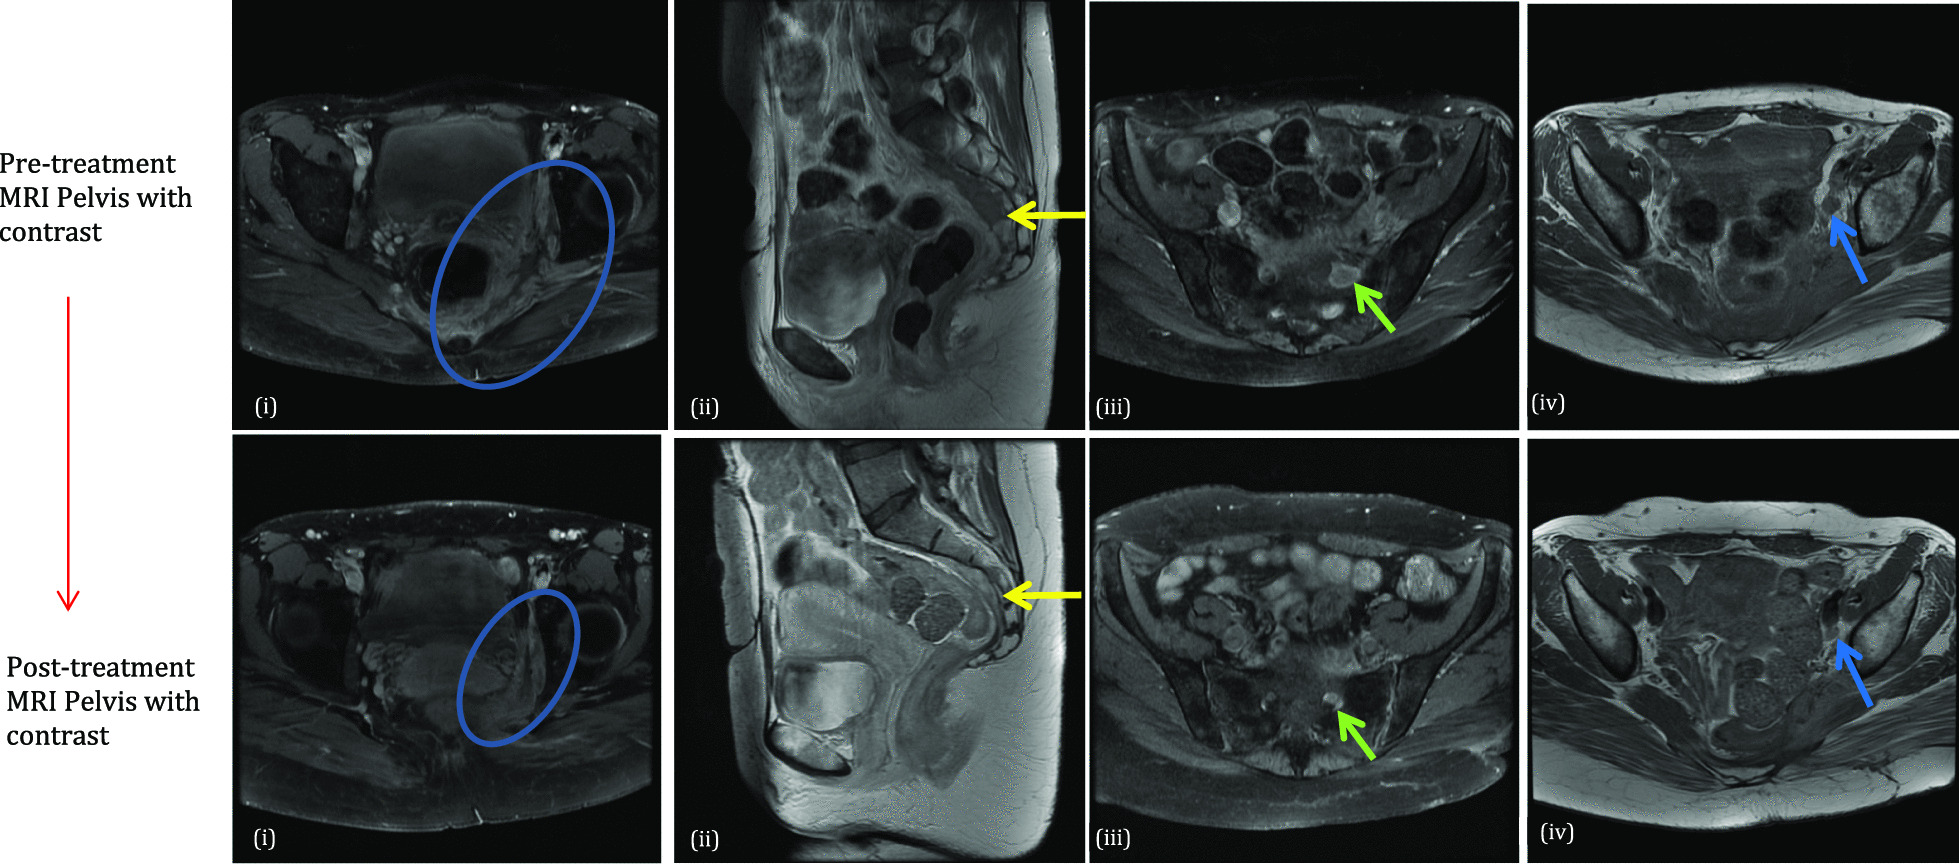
Magnetic resonance imaging scans of the pelvis obtained before and after her initial course of treatment. (i) Axial post-contrast fat-saturated T1WI and (ii) Sagittal T2WI show a significant reduction in the volume of soft tissue within the left hemipelvis (blue circles) and presacral space (yellow arrows). (iii) Axial post-contrast fat-saturated T1WI shows residual but less extensive enhancing soft tissue along the sacral nerve roots (green arrows). This is seen to continue to involve the exiting nerve roots, lumbosacral plexus and sciatic nerve—the thickness of these are, however, reduced. (iv) Axial T2WI shows reduced-volume left iliac chain lymphadenopathy (blue arrows)
